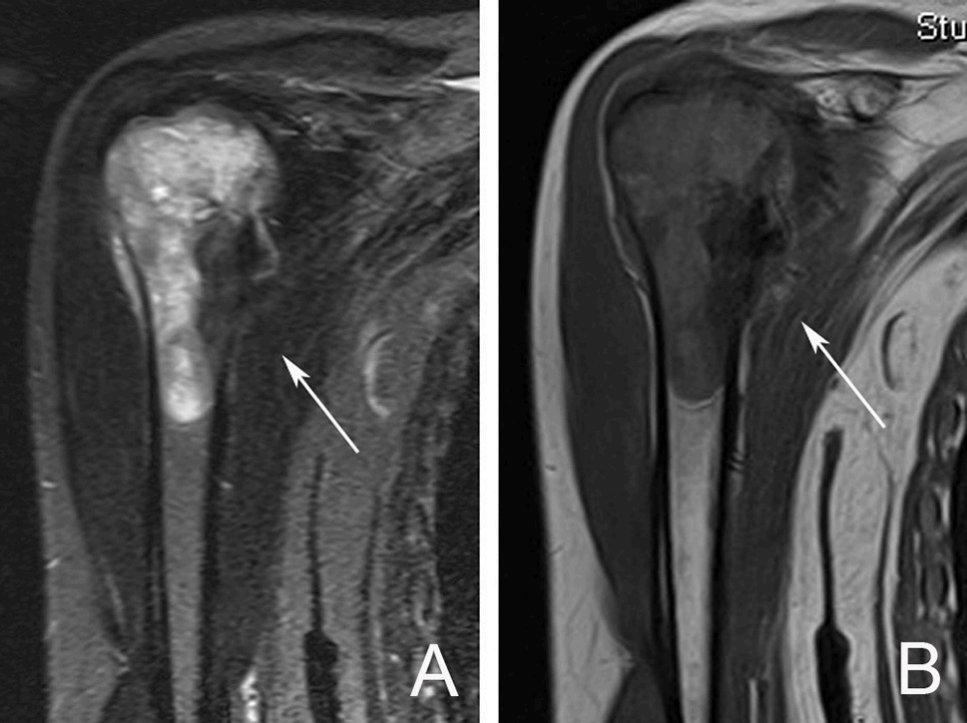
MRI scan demonstrating a right proximal humerus metastatic lesion. (A) T2 weighted MRI sequence showing the extent of intramedullary involvement (arrow). (B) T1 weighted MRI sequence showing complete involvement of the proximal humerus with cortical breach at the medial calcar region (arrow).
